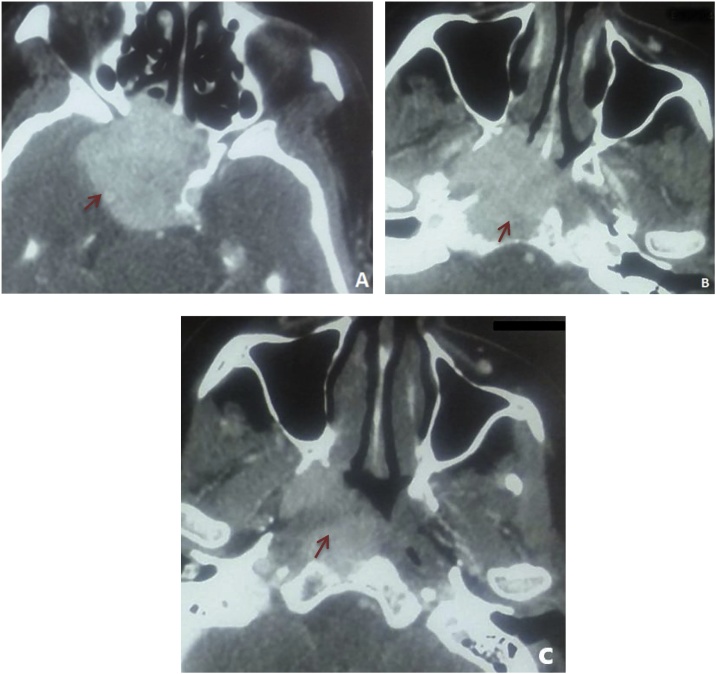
CT scan images showing the tumor of the sella turcica, extending to the sphenoid sinus (A), the nasopharynx (B) and the para-pharyngeal space (C).
